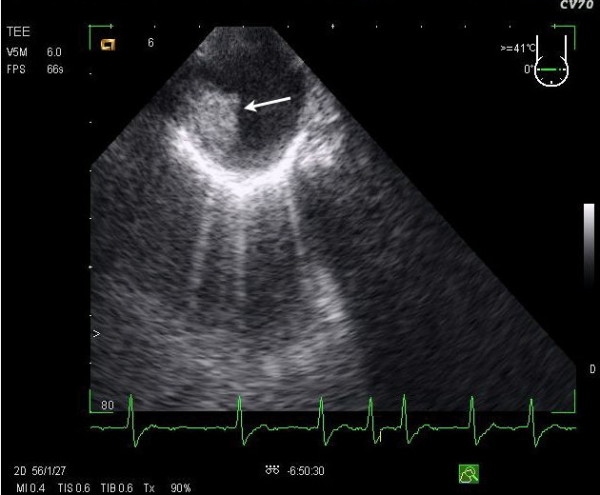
A trans-esophageal echocardiographic scan showing an intra-luminal pyramidal lesion 1.4 cm×1.5 cm in size with an irregular surface on the anterior wall of the descending aorta (arrow).
